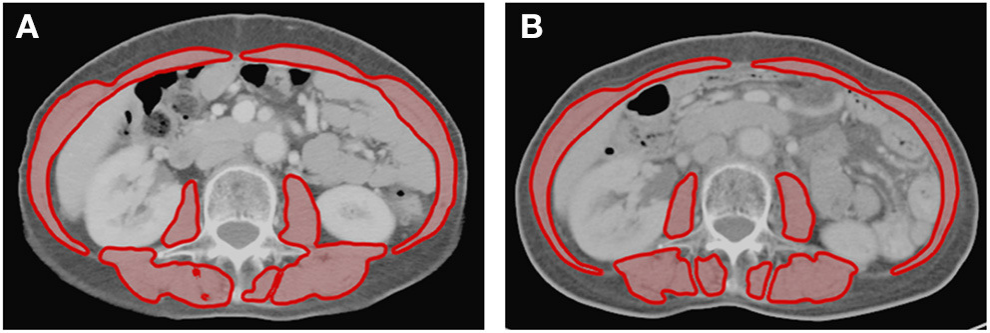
Axial cross-sectional areas of skeletal muscle (red) on CT images at the L3 vertebral level (A) before and (B) after treatment in one patient. The skeletal muscle areas of this patient were 81.6 and 77.3 cm^2^ before and after treatment, respectively. This patient had a reduction of 5.3% of skeletal muscle after treatment.
